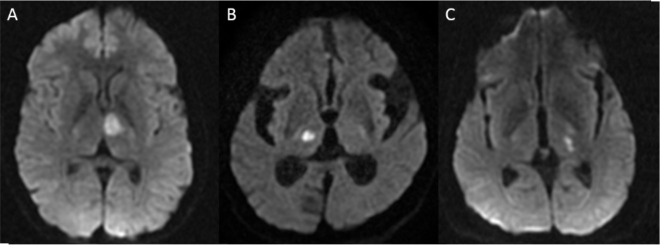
The variative territories of thalamus on DWI: anteromedian (A), central (B), and posterolateral (C).
